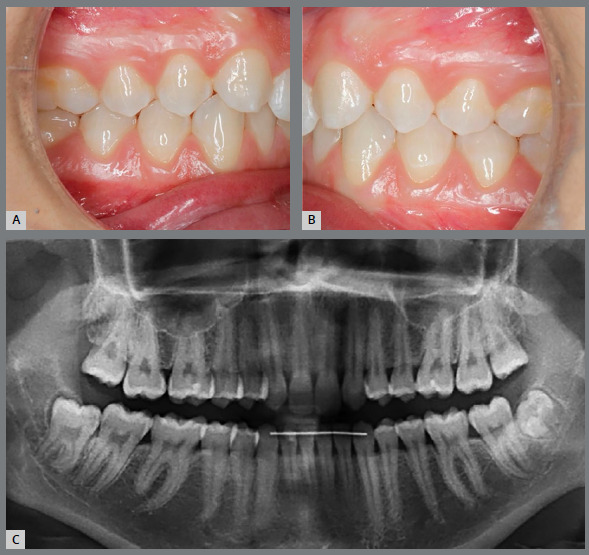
A, B) Posterior occlusion on the right and left sides, periodontal health in the premolar region with 7-year follow-up. C) Follow-up panoramic radiograph.
